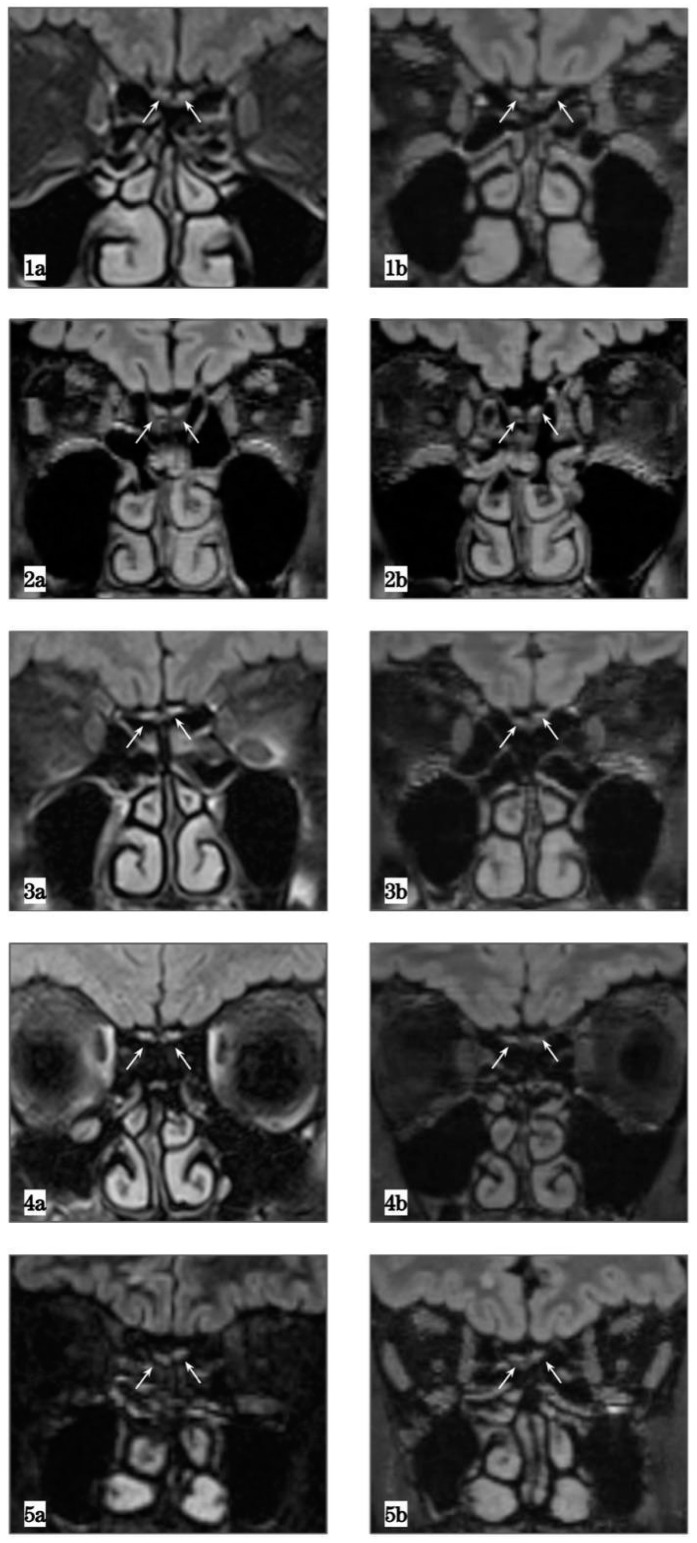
Magnetic resonance imaging (MRI) showing radiological findings of the included cases: four hyposmic patients (1–4) and one control (5). Frames (F.) (1a–5a) are pre-COVID-19 MRIs, showing normal olfactory bulb (OB) volumes. F (1b–5b) represent post-COVID-19 MRIs of the same patients revealing reduction in OB volume in hyposmic patients and unchanged OB in the subject without smell disorder (5b). All captures are isotropic FLAIR 3D (matrix 288, FOV 245, 0.9 mm, TR 8500, TE 386) sequences.
